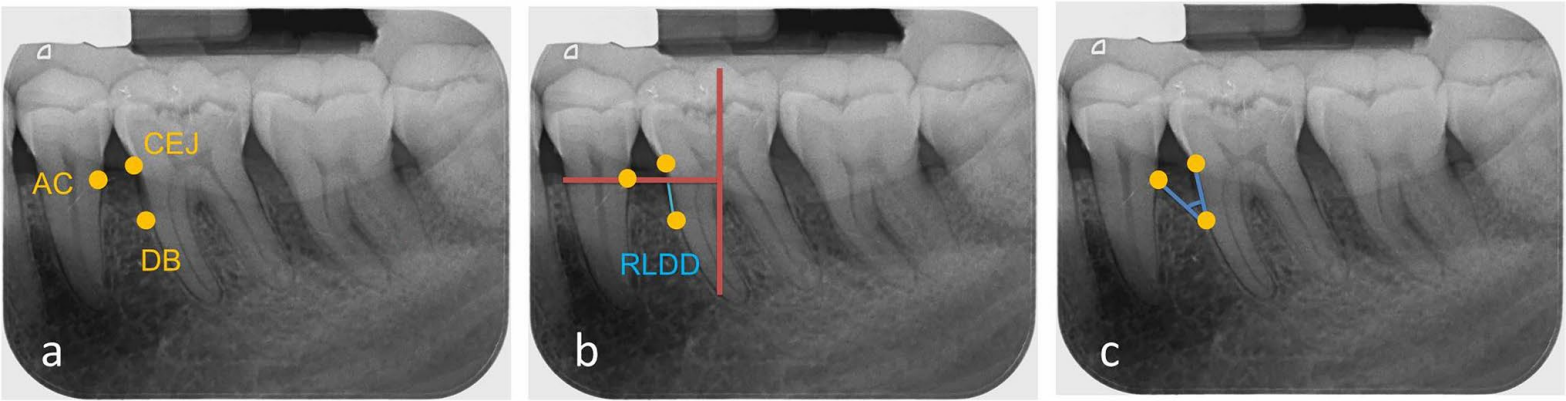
Intrabony defect radiographic measurements. a Reference point identification: cemento-enamel junction (CEJ), alveolar crest (AC), and defect base (DB). b Reference line identification (in red): vertical line corresponding to long axis and horizontal perpendicular line passing through AC and identifying radiographic linear defect depth (RLDD) in blue. c Radiographic angle connecting CEJ, DB, and AC
